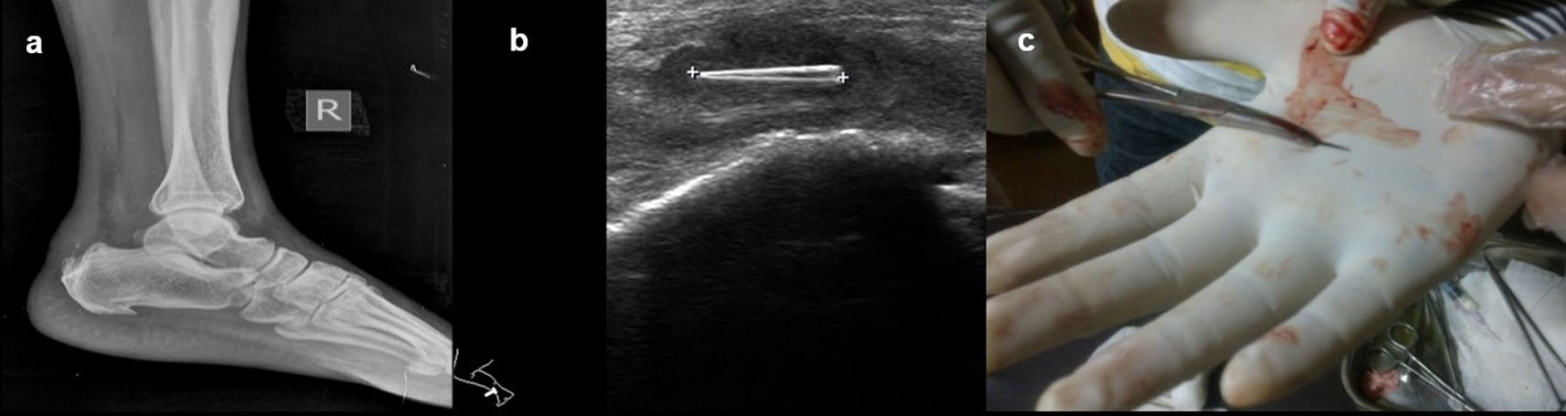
a–c: A radiograph of the lateral view of the right foot revealed calcific tendinitis of the Achilles tendon with thickening of the Kager fat pad and fat stranding (a). USG revealed that a well-defined thorn visualized in the Achilles tendon with associated changes of tendinitis (b). Thorn removed under ultrasound guidance (c).
